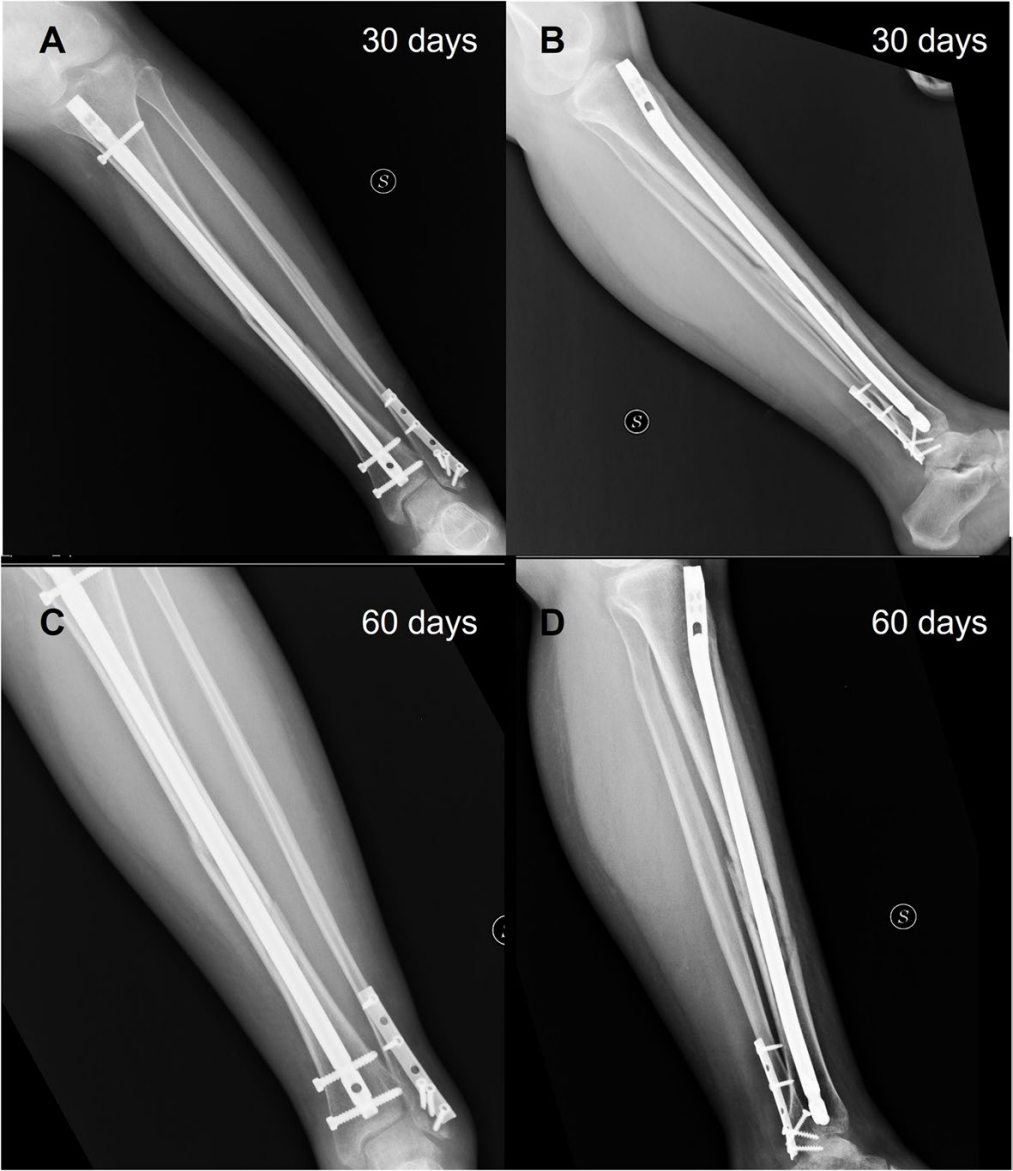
Control plain radiographs at 30 days postop. (A and B) and at 60 days follow-up (C and D)
